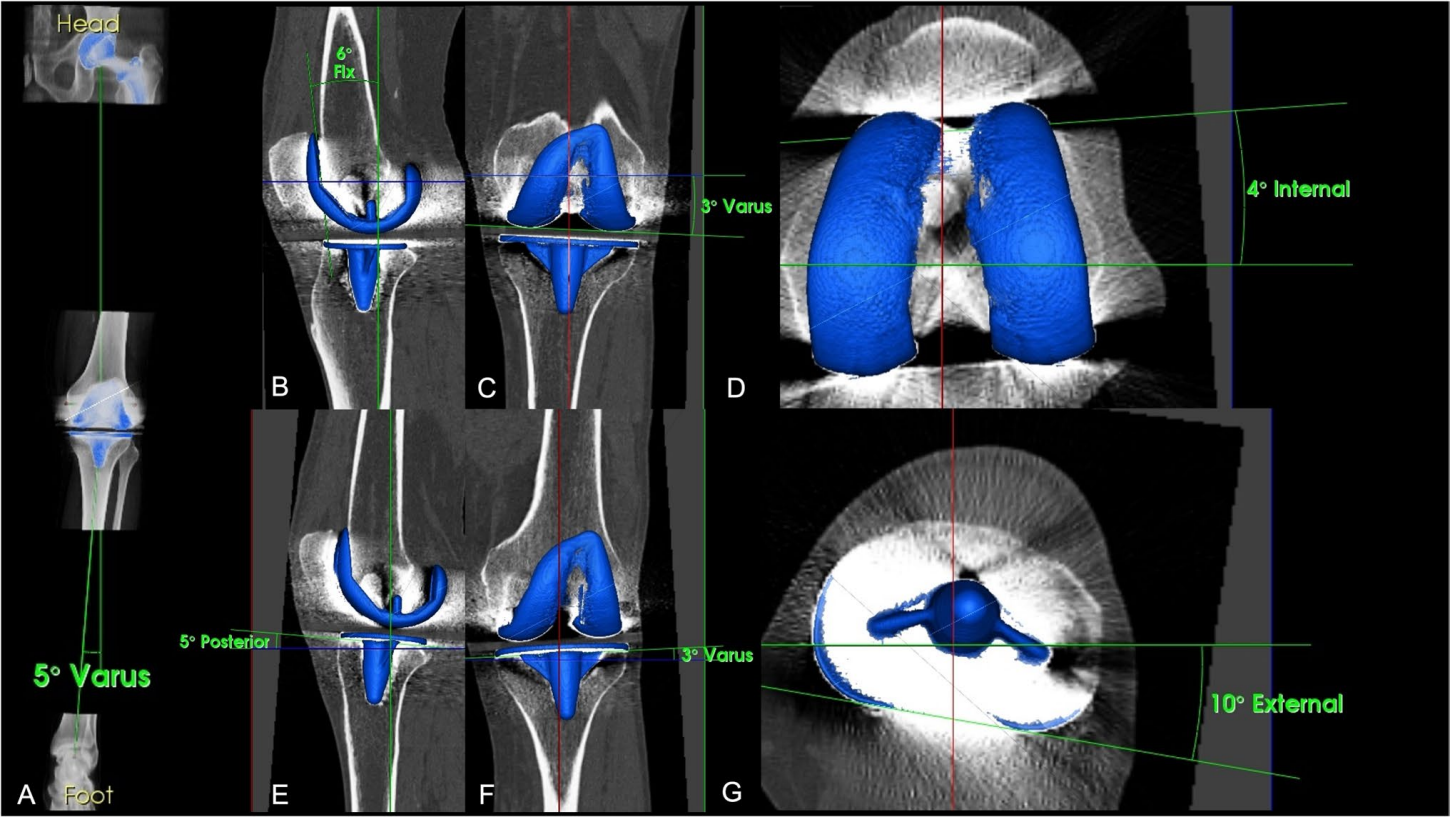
SPECT/CT images of a 74-year-old male patient with left painful total knee arthroplasty (TKA). Mechanical alignment and TKA position were assessed using a customized validated 3D-software “Orthoexpert®”. a–g show angle measurements of the femoral (b–d) and tibial (e–g) component. The increased internal rotation of 4° of the femoral shield (d) and varus positioning of 3° of the tibial component (f) causes pain in this TKA patient
